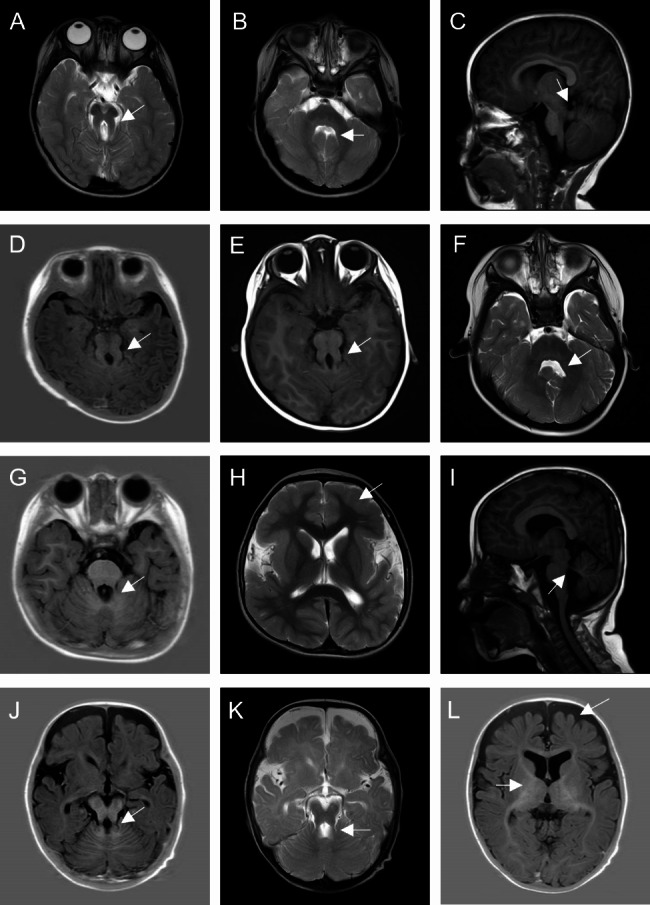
MRI results of four patients. (A-C) Patient 2.(A) Axial T2-weighted image shows cerebellar vermis hypoplasia, a deepened interpeduncular fossa and thickened, elongated superior cerebellar peduncles (arrow), presents a “molar tooth sign”; (B) Enlargement of the fourth ventricle, “bat-wing”(arrow); (C) Parasagittal T1-weighted image showed thickened, elongated, and horizontally oriented superior cerebellar peduncles (arrow); (D-F) Patient 3. (D) Axial T1-weighted image (7 months) shows a blurry deepened interpeduncular fossa and thickened, elongated superior cerebellar peduncles (arrow); (E) Axial T1-weighted image (3 years) shows vermian hypoplasia, thickened, elongated superior cerebellar peduncles (arrow); (F) Axial T2-weighted image (3 years) shows a “bat-wing” of the fourth ventricle; (G-I) Patient 4. (G) Axial T1-weighted image shows slight vermian dysplasia and thin but elongated cerebellar peduncles (arrow); (H) Axial T2-weighted image shows thickened cortex, sparse gyri, and shallow sulci especially in the bilateral frontotemporal lobes (arrow); (I) Parasagittal T1-weighted image shows cerebellar hypoplasia, thin, elongated and horizontally oriented cerebellar peduncles (arrow); (J-L) Patient 4. (J-K) Axial T1 and T2-weighted image shows thin and elongated cerebellar peduncles (arrow); (L) The subarachnoid space is widened bilaterally in the frontotemporal regions (arrow), the ventricular system is enlarged, and the volume of white matter is less than peers, suggesting white matter dysplasia
